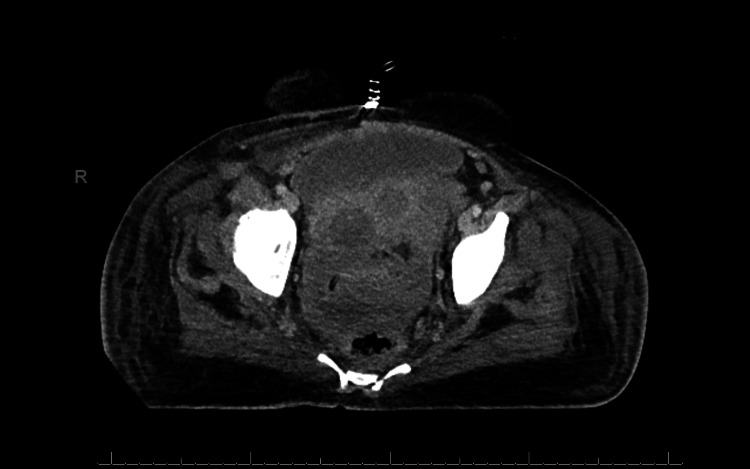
CT scan shows a large Pelvic heterogeneous soft tissue density mass.
